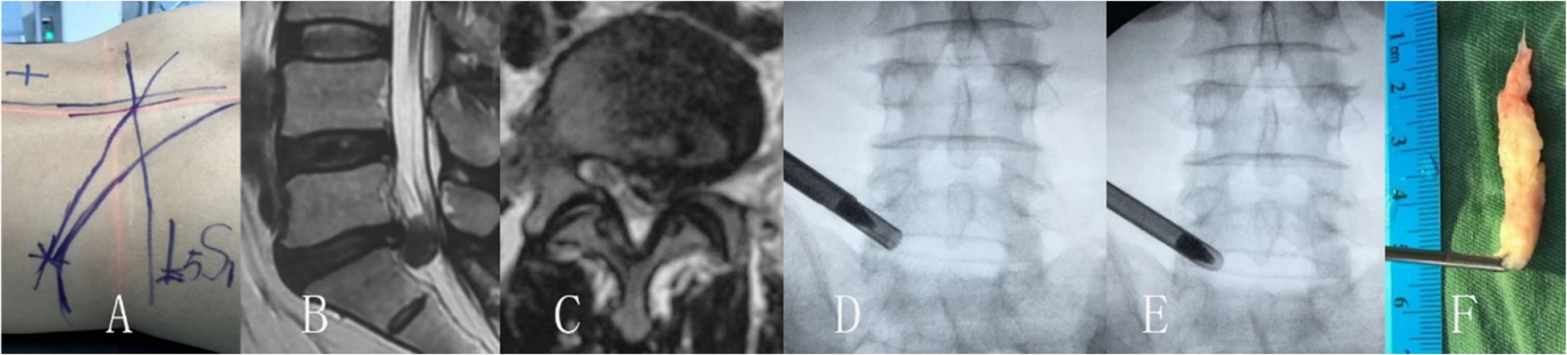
Male patient of 41 years old had L5/S1 paracentral disc herniation and left leg pain combine with numbness. The leg pain was disappeared after transforaminal endoscopic lumbar discectomy and nerve decompression, but the numbness was stayed until 6 months after surgery. a show the skin marks, b and c was the MR image of the left paracentral disc herniation, d and e was the image of the endoscopic procedures, and F was the removed disc herniation fragment
